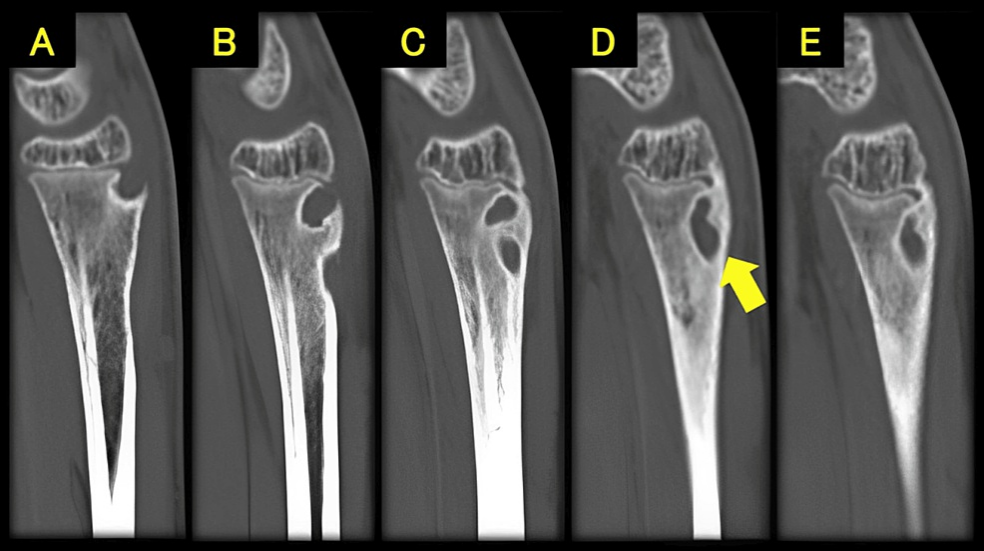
Computed tomography scans (sagittal view) showing a radiolucent area in the distal radius (yellow arrow) Sagittal view: from A to E, ulnar to radial
